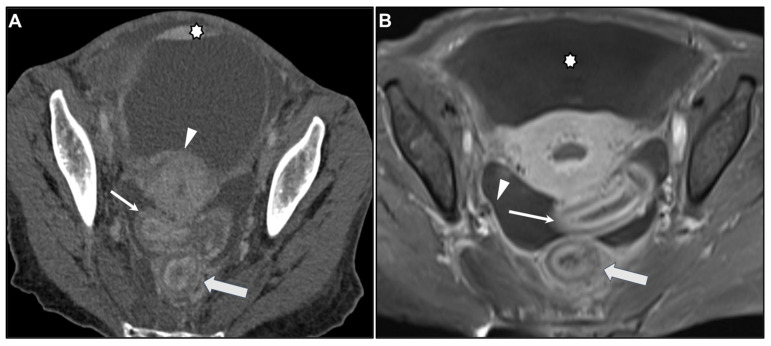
Concomitant peritoneal carcinomatosis (PC). (A) Axial contrast-enhanced CT. (B) MR image: Axial CE-GRE-T1WI. Linitis plastica-like metastases from invasive lobular carcinoma involving the rectum (thick arrows) and sigmoid colon (thin arrows). Concomitant PC is demonstrated by deposits over the bladder (* in (A)) and around the uterus (arrowhead in (A)), with ascites (* in (B)) and enhancing parietal peritoneum (arrowhead in (B)).
